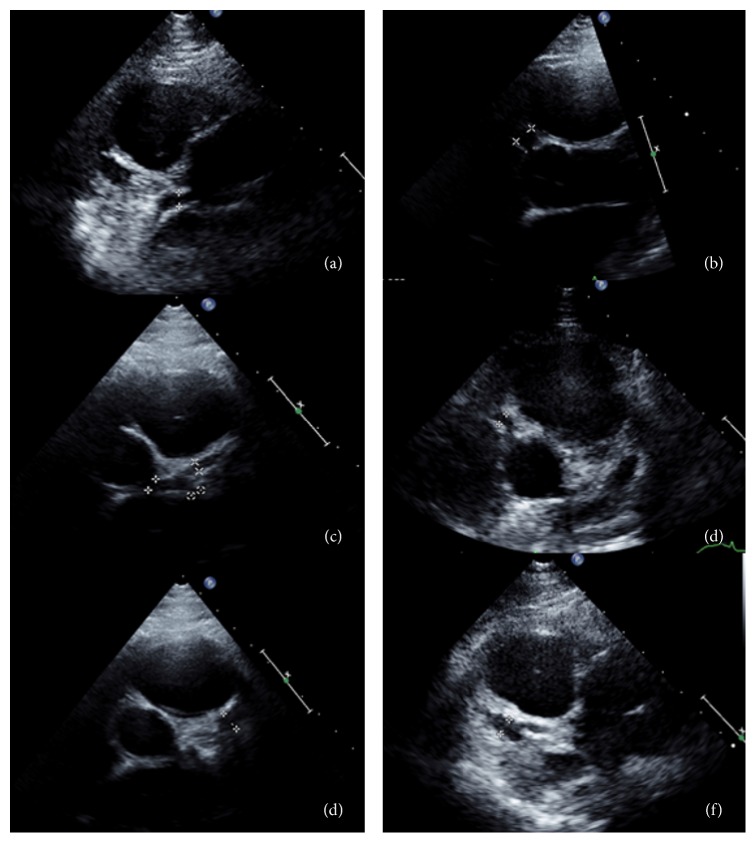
Echocardiographic images demonstrating coronary artery ectasia. Parasternal short axis views from echocardiogram. (a) Left coronary artery measured at proximal end. (b) Right coronary measured at proximal end. (c) LCA measured at distal end. (d) RCA measured at proximal end. (e) LCA at bifurcation into the left circumflex and left anterior descending coronary. (f) RCA measured at the distal end.
